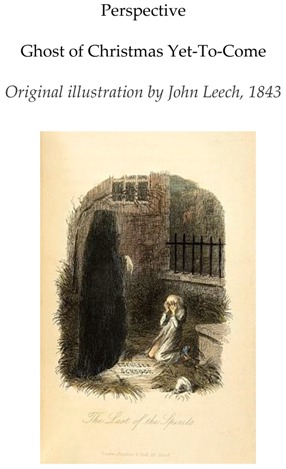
As one year closes and another begins, I find myself reflecting on ovarian cancer diagnostics. It is truly humbling how little we have accomplished in this field over the last half-century. All the while, the rest of the world has been busy. Since the first biomarker was reported, we have harnessed the atom and ushered in the Nuclear Age. Since the first *ovarian* biomarker was reported, we have invented the integrated circuit and spawned the dynamic Information Age. Yet, as gynecologic oncologists, we continue to struggle with the early identification of ovarian cancer and whether ovarian cancer actually begins in the ovary at all. The first serum biomarker for epithelial ovarian cancer was introduced in 1965 (carcinoembyonic antigen, CEA) [1]. This was a milestone in cancer diagnostics, as prior to this, oncologists were equipped with little to detect or monitor ovarian cancer. Keep in mind that this was when ultrasound was just emerging as a very rudimentary medical diagnostic instrument, and well before the advent of computed tomography (CT) or magnetic resonance imaging (MRI). Now some fifty years later, it is easy to ask, “Why haven’t we done more?” Perhaps the recent focus on molecular-genetic technology and personalized cancer treatment will inspire a new Diagnostic Age in oncology. I am an optimist at heart, and am hopeful that our biomarker story will read somewhat like the Charles Dickens novella, A Christmas Carol, where the return of Jacob Marley’s ghost 7 years after his death helps give clarity to the past, present, and yet-to-come.
